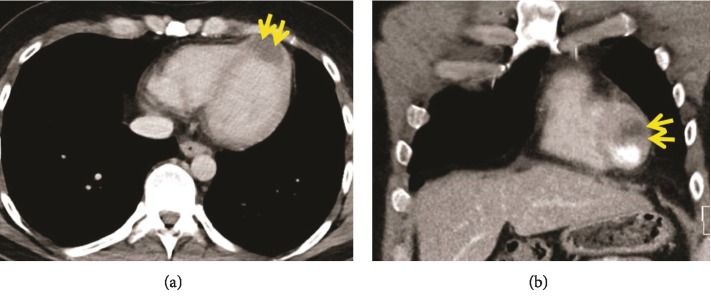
(a, b) Chest computed tomography with contrast shows a mass in the apex of the left ventricle (arrows).
